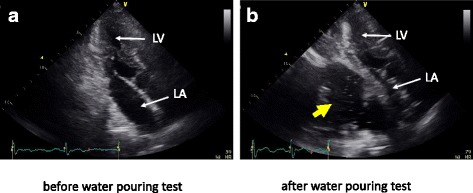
Transthoracic echocardiography. a Echocardiography before the water pouring test. b Echocardiography showed that after instillation of 2000 ml of saline, the left atrium was markedly compressed and the area of the mitral annulus was reduced. The yellow arrow indicates saline in the stomach
